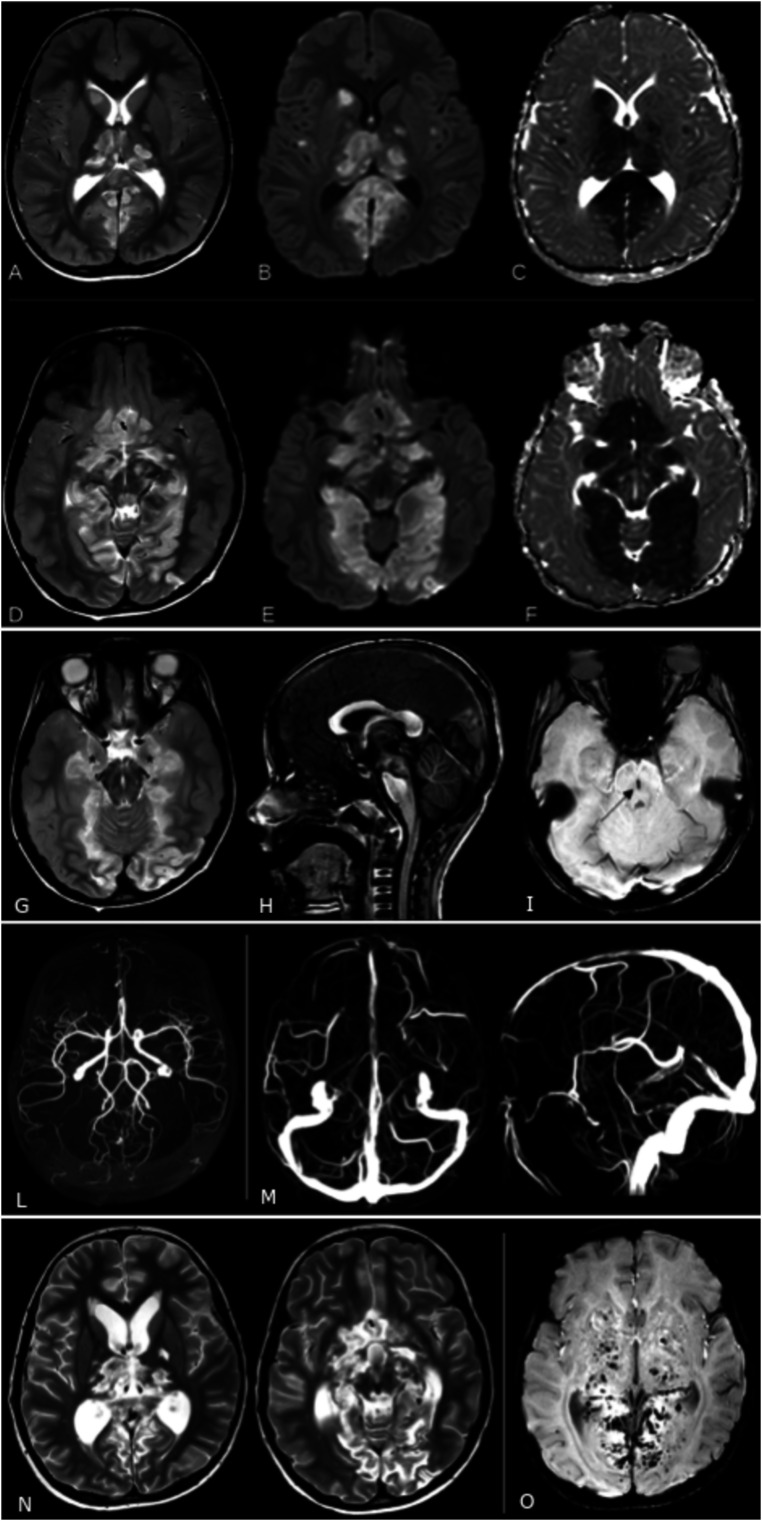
Axial (a, d) TSE T2, (b, e) DWI and (c, f) ADC sequences showing the presence of large areas of T2 hyperintensity and restricted diffusion consistent with striking parenchymal ischemia. (g) Axial TSE T2 sequence showing mild bilateral uncal herniation (asterisks) and effacement of the perimesencephalic cisterns. (h) Sagittal 3D TFE T1 sequence showing a sagging brainstem with midbrain descent through the tentorial incisura. (i) Axial SWI sequence showing a midbrain sagittal hypointense stripe (arrow) consistent with the presence of hemosiderin components (Duret’s hemorrhage). (l) 3D time of flight angiography and (m) phase contrast venography showing no abnormalities with the regular representation and patency of the main arterial and venous intracranial vessels. Bottom row: follow-up MRI performed 30 days after admission. (n) Axial TSE T2 sequence showing the malacic evolution of the parenchymal lesions and the dilatation of the ventricular system. (o) Axial SWI sequence showing the presence of multiple microhemorrhages.
